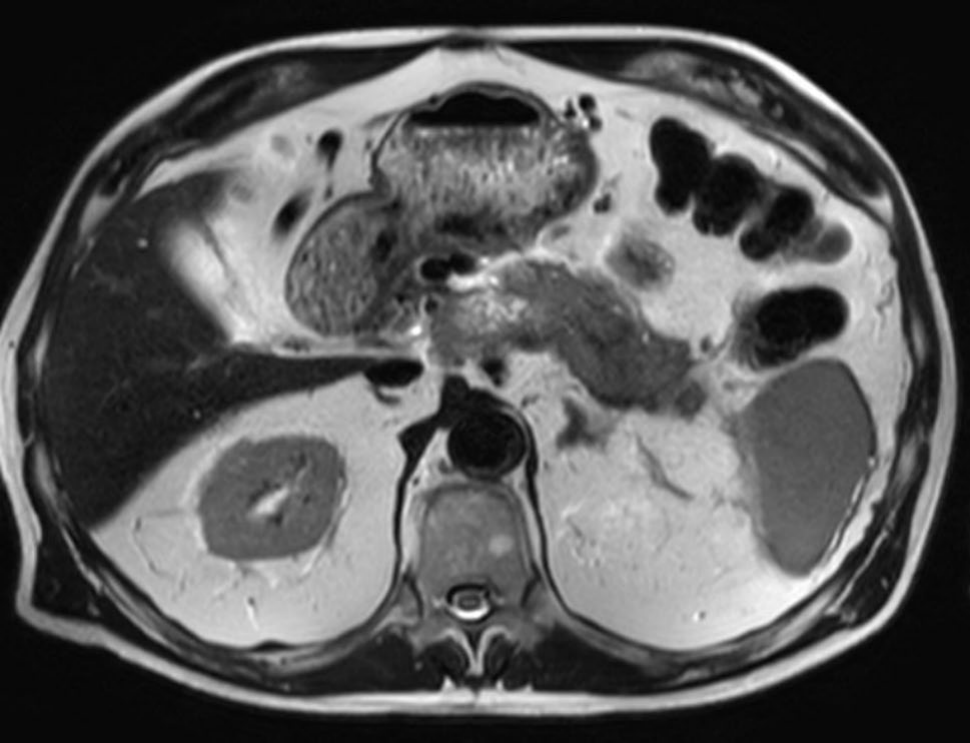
MRI done 2 weeks after initial CT scan, axial T2 sequence. Extensive peripancreatic phlegmonous changes with fluid extending toward the splenic hilum
